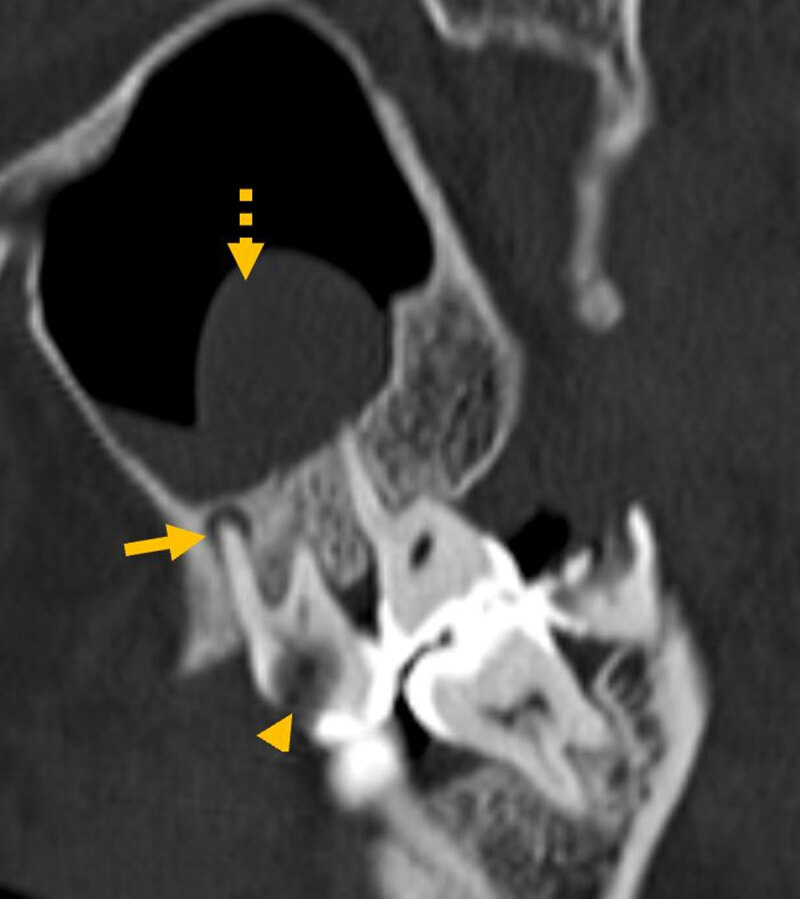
Dental caries (arrowhead) and periapical lucency (arrow) in the same tooth with adjacent polypoidal mucosal thickening (dashed arrow).
